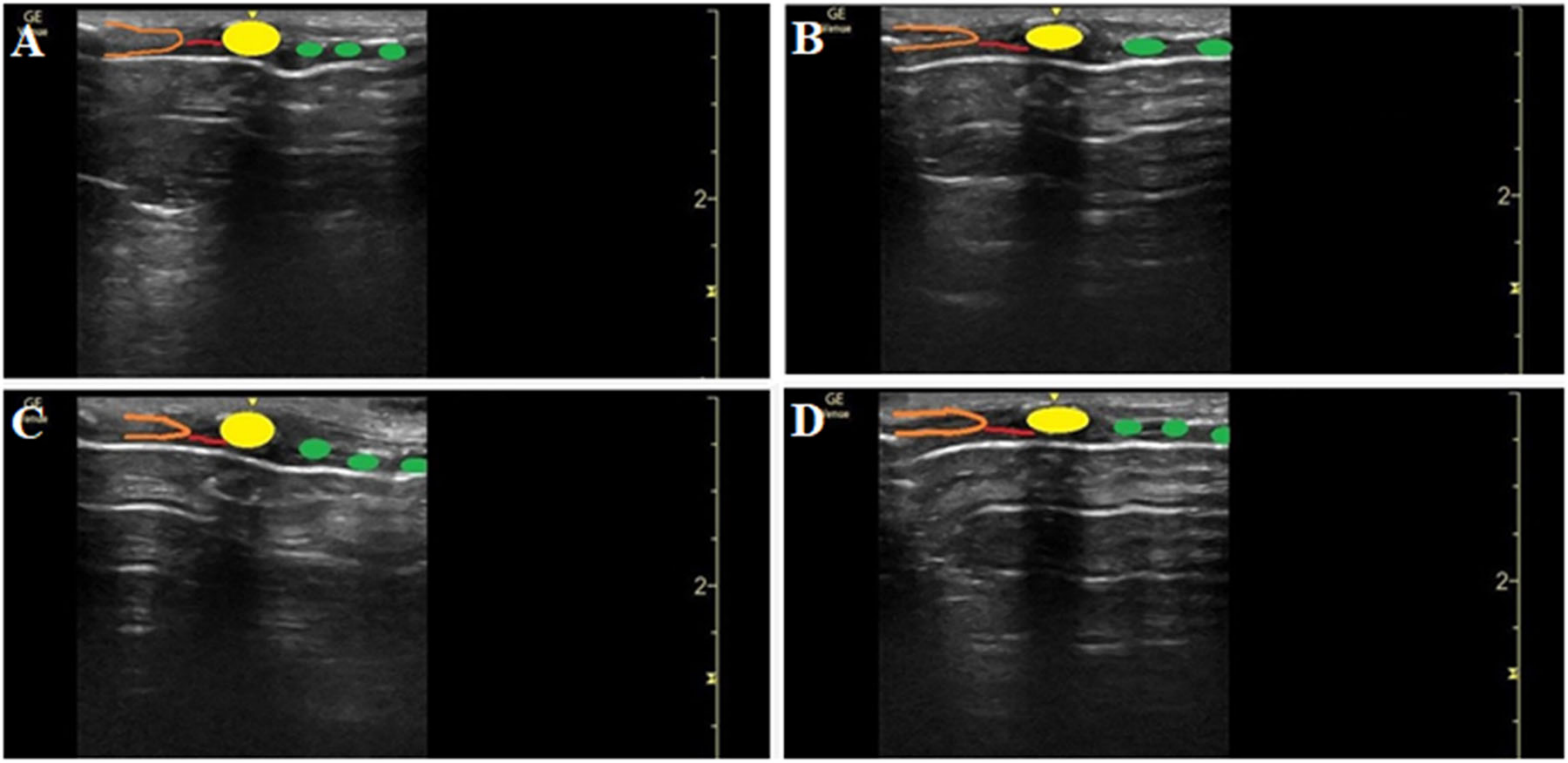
Longitudinal ultrasound view of the trachea while using ETT (A), I‐gel (B), LarySeal (C), and LMA (D). Longitudinal midline‐scan view of the neck shows the cricothyroid membrane in red. Orange, yellow, and green represent the thyroid cartilage, the cricoid cartilages, and tracheal rings, respectively.
